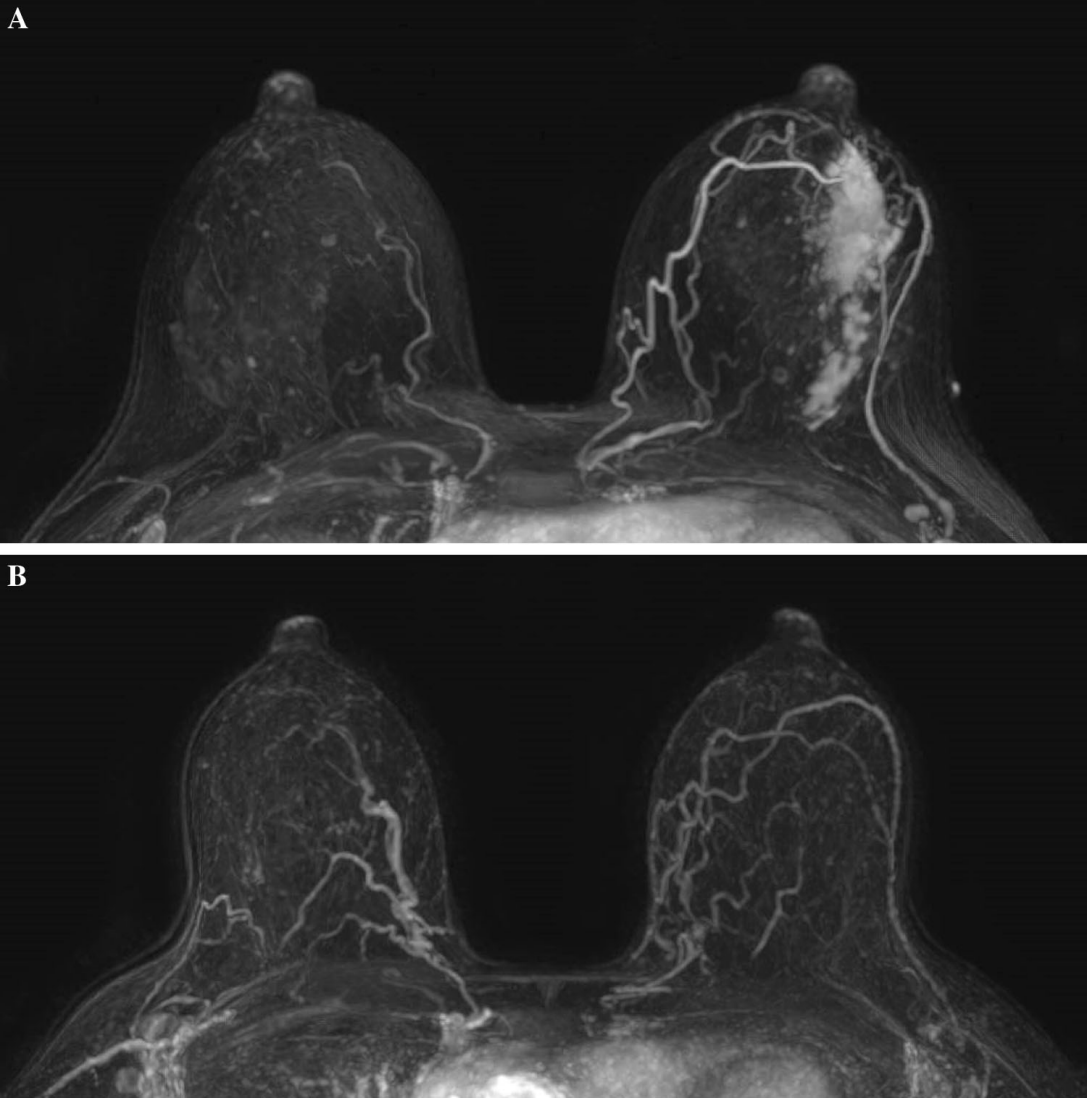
Radiological complete response on dynamic contrast-enhanced MRI after neoadjuvant systemic therapy. Breast MRI in a patient with left-sided breast cancer before the start of neoadjuvant systemic therapy (A) and after neoadjuvant systemic therapy (B). Maximum intensity projection (MIP) images after treatment show no pathologic enhancement in the left breast, radiologically assessed as a complete response
